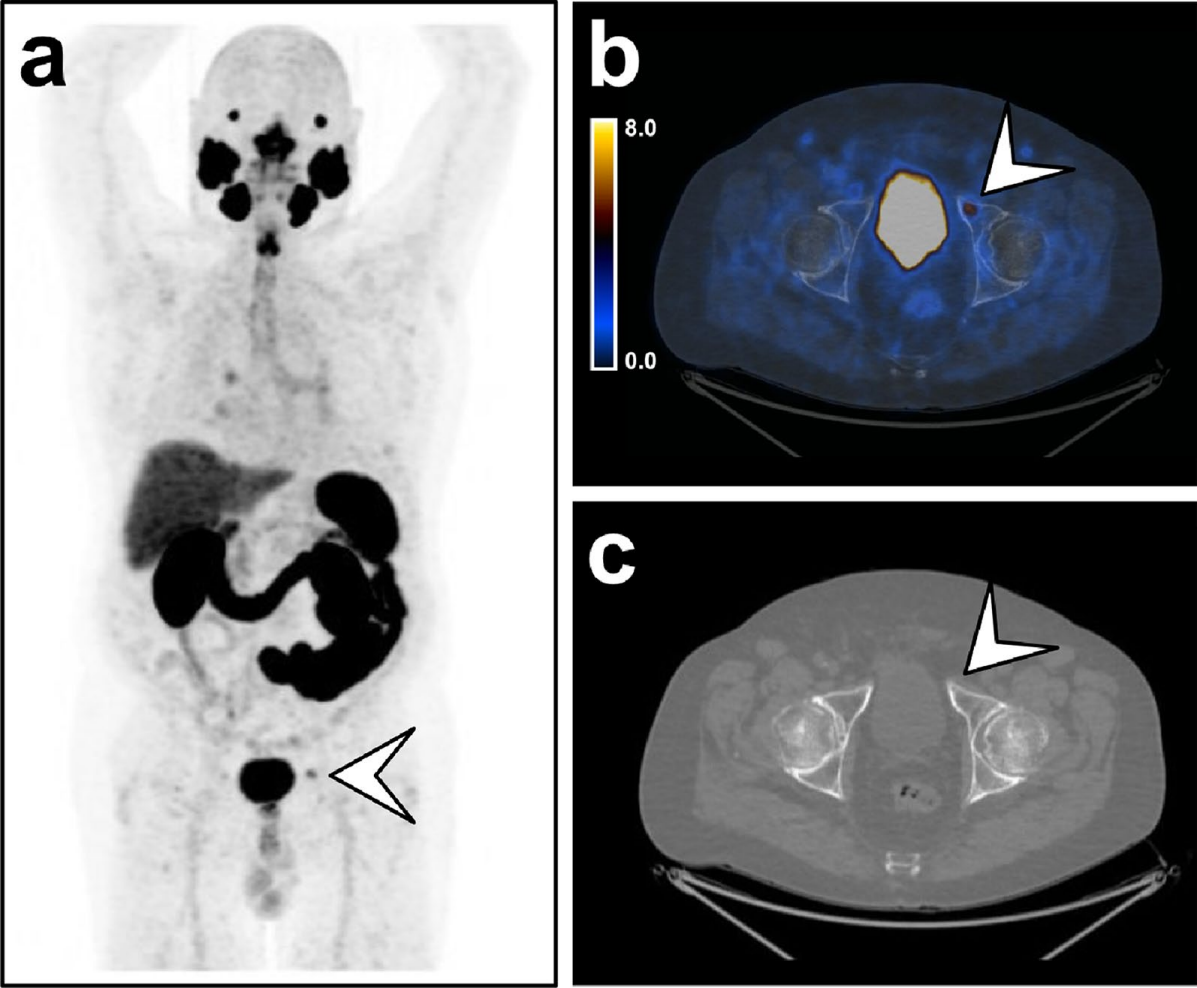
60-year-old male with BCR of PCa underwent [^68^Ga]Ga-PSMA PET/CT for BCR (PSA 3.6 ng/ml) (a) Whole-body maximum intensity projection (MIP) image shows a PSMA positive pelvic lesions on the left side (white arrowhead) (b) Axial fused [^68^Ga]Ga-PSMA PET/CT shows a lesion in the left anterior acetabular column with a SUV_max_ of 5.3 (white arrow head). (c) A CT scan did not reveal any sclerotic morphological correlates (white arrowhead). The lesion was classified as a PSMA-RADS 3B lesion and was rated as malignant. Following radiotherapy of the pelvic lesion, the PSA level normalized and the lesion was confirmed as a true positive bone metastasis
